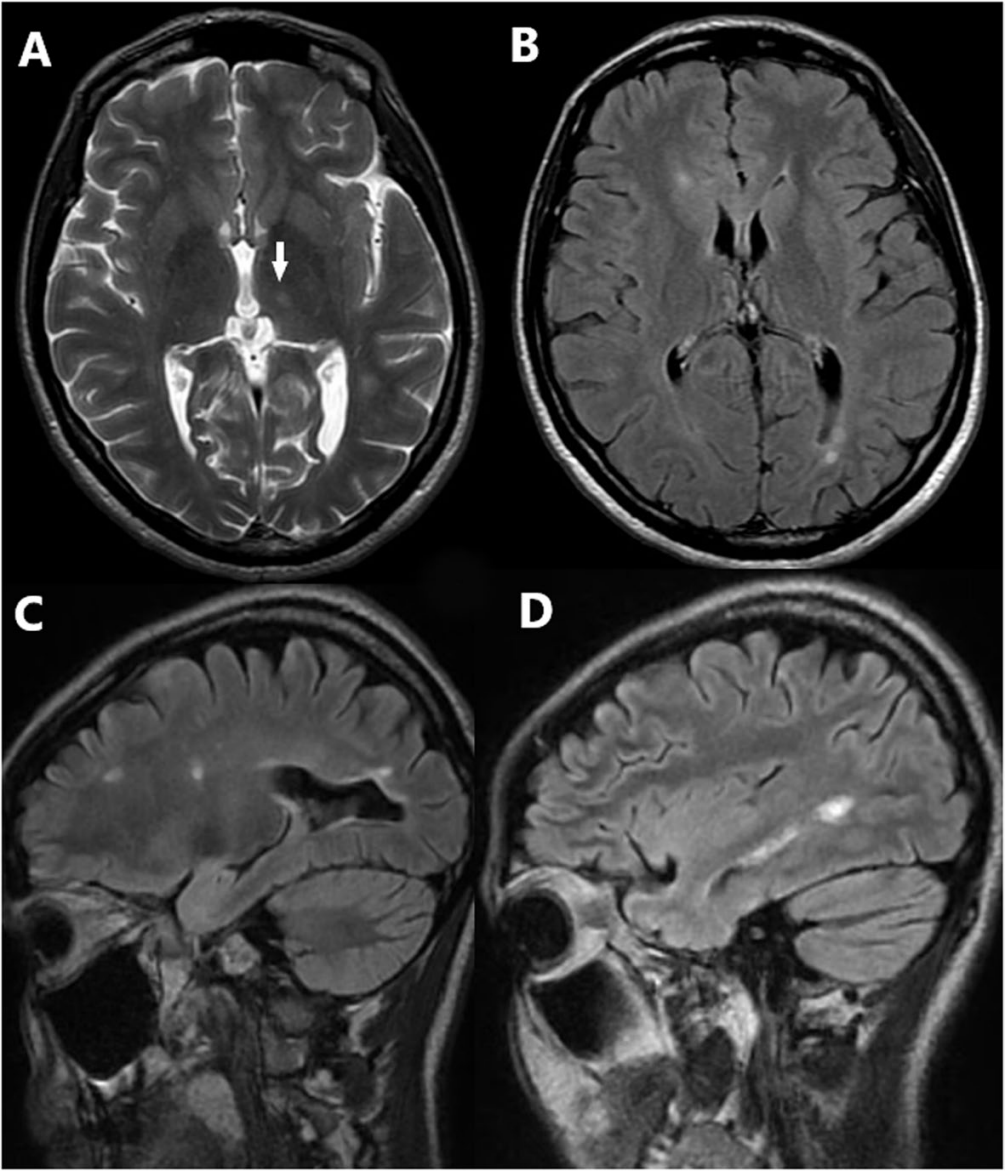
Brain MRI scans upon first admission. Axial T2 scan (A) shows a lesion in the left thalamus (full white arrow). Axial FLAIR (B) and sagittal FLAIR CUBE with gadolinium contrast media (C-D) scans reveal multiple periventricular white matter lesions typical for MS including a gadolinium enhancing lesion in the left occipital area, as well as a juxtacortical lesion in the left frontal region
